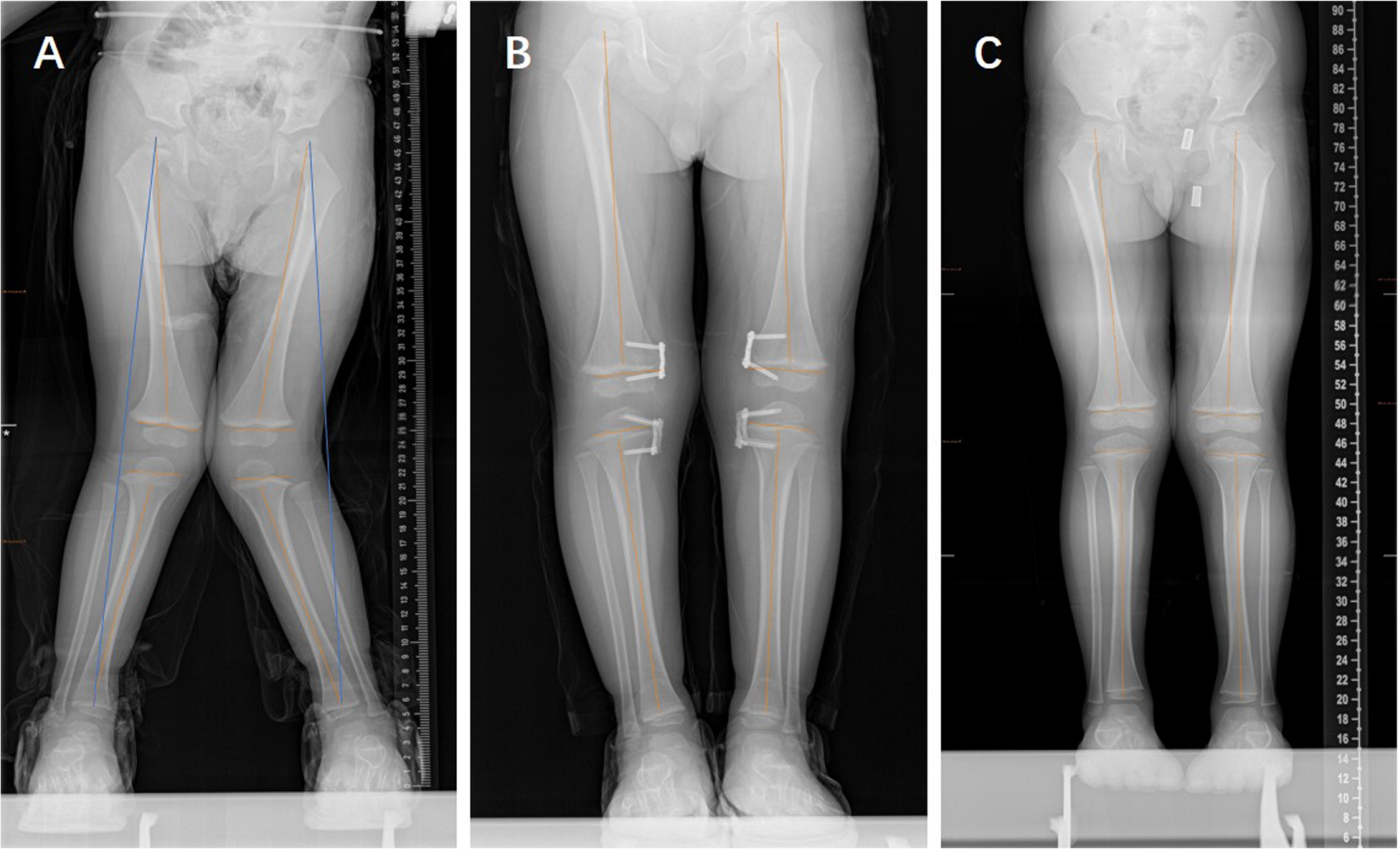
Comparison of full-length anteroposterior standing radiographs preoperatively, before removing the inner fixation device and at the last follow-up in a 2-year-old boy with bilateral idiopathic genu valgus deformities. a, pre-operation; b, before removing the inner fixation device (6 months TH surgery); c, the last follow-up (22 months after removing the inner fixation device)
